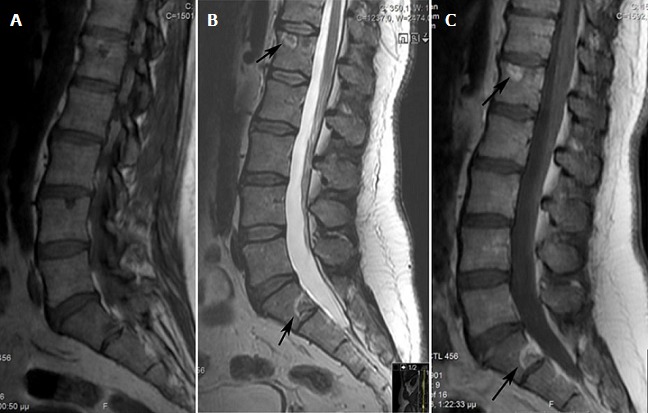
MRI of the lumbar spine, sagittal section, T1: (A) T2; (B) and gadolinium-enhanced T1-weighted; (C) sequences: presence of nodular bone metastases with a halo (arrows)
